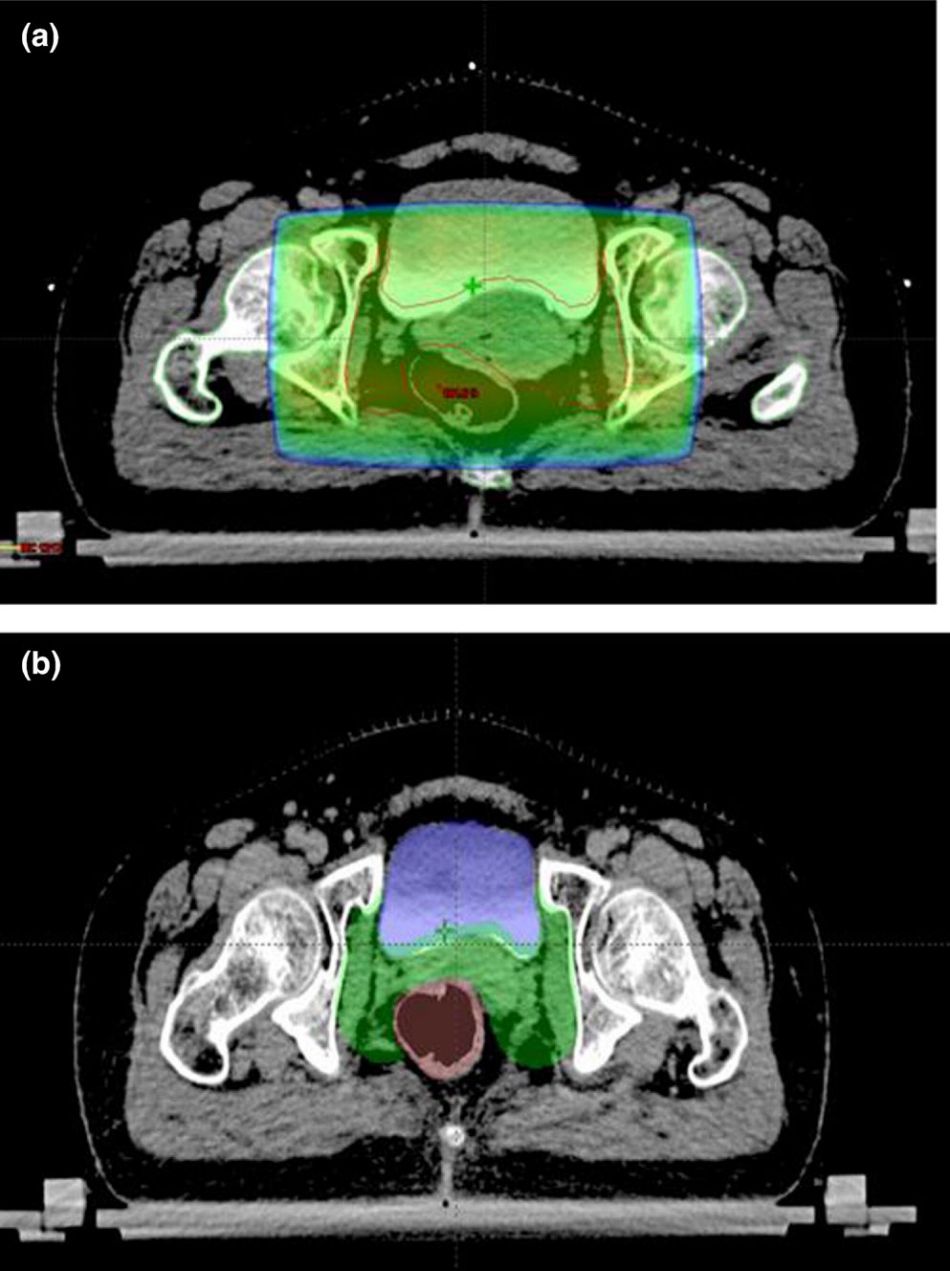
Computed tomography images showing radiotherapy planning using: (a) conventional four‐field box technique; and (b) IMRT planning. Normal tissues such as bladder and bowel are relatively spared in IMRT planning. *Note:* IMRT, intensity modulated radiation therapy.
